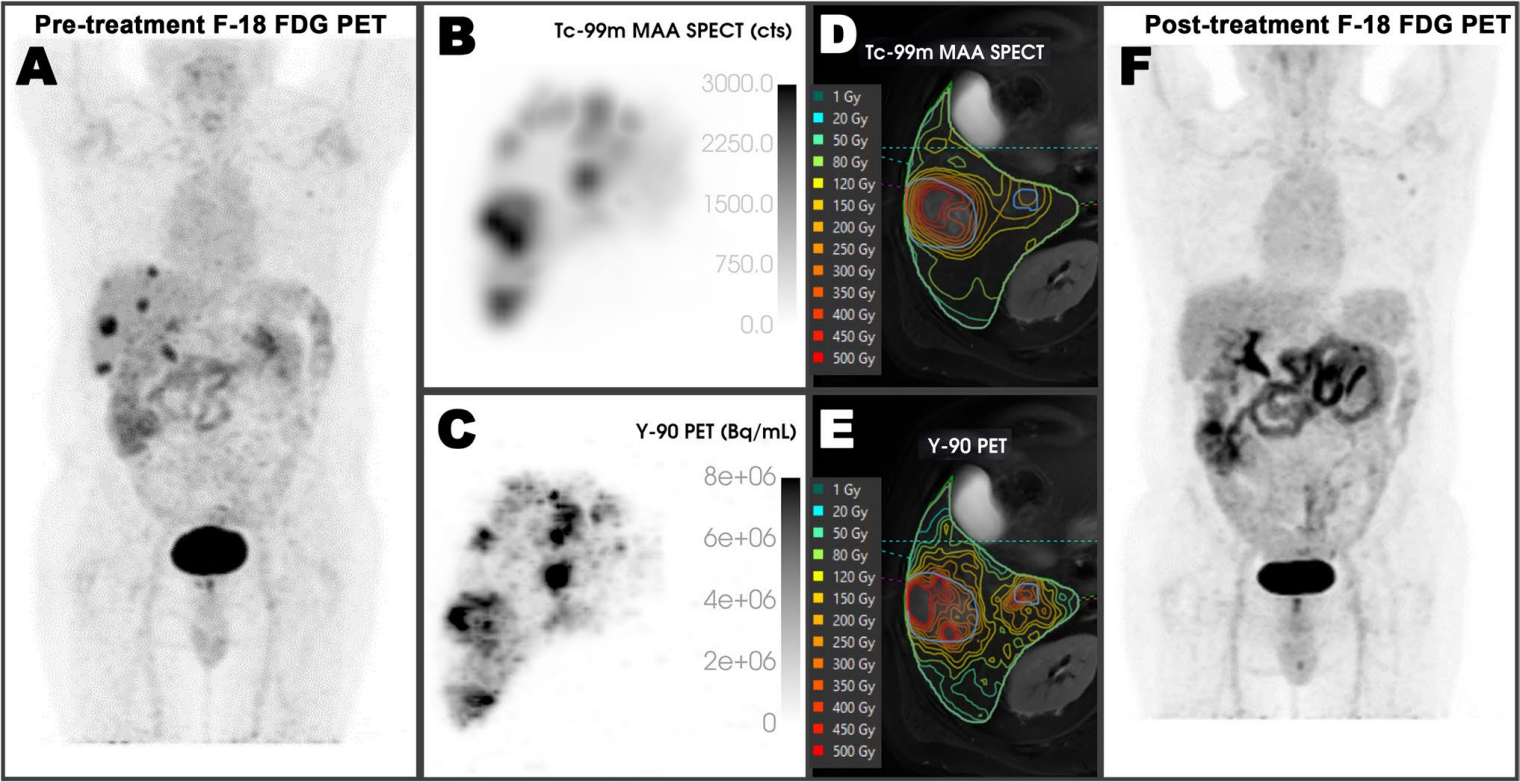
A 67-year-old man underwent treatment for multiple metastases of colorectal carcinoma in the liver with 2.80 GBq Y-90 glass microspheres. Intense accumulation of Tc-99m-MAA in the tumors was observed in both pre-treatment SPECT MIP images (B) and Tc-99m-MAA SPECT/CT -MRI fusion images (D). Similarly, post-treatment PET MIP (C) and Y-90 PET/MRI fusion images (E) also revealed intense uptake of Y-90 microspheres in the tumors. The mean radiation dose to tumors was calculated as 179.80 Gy with pre-treatment SPECT and 273.2 Gy with post-treatment PET. On follow-up ^18^F-FDG PET imaging complete response was observed (F)
